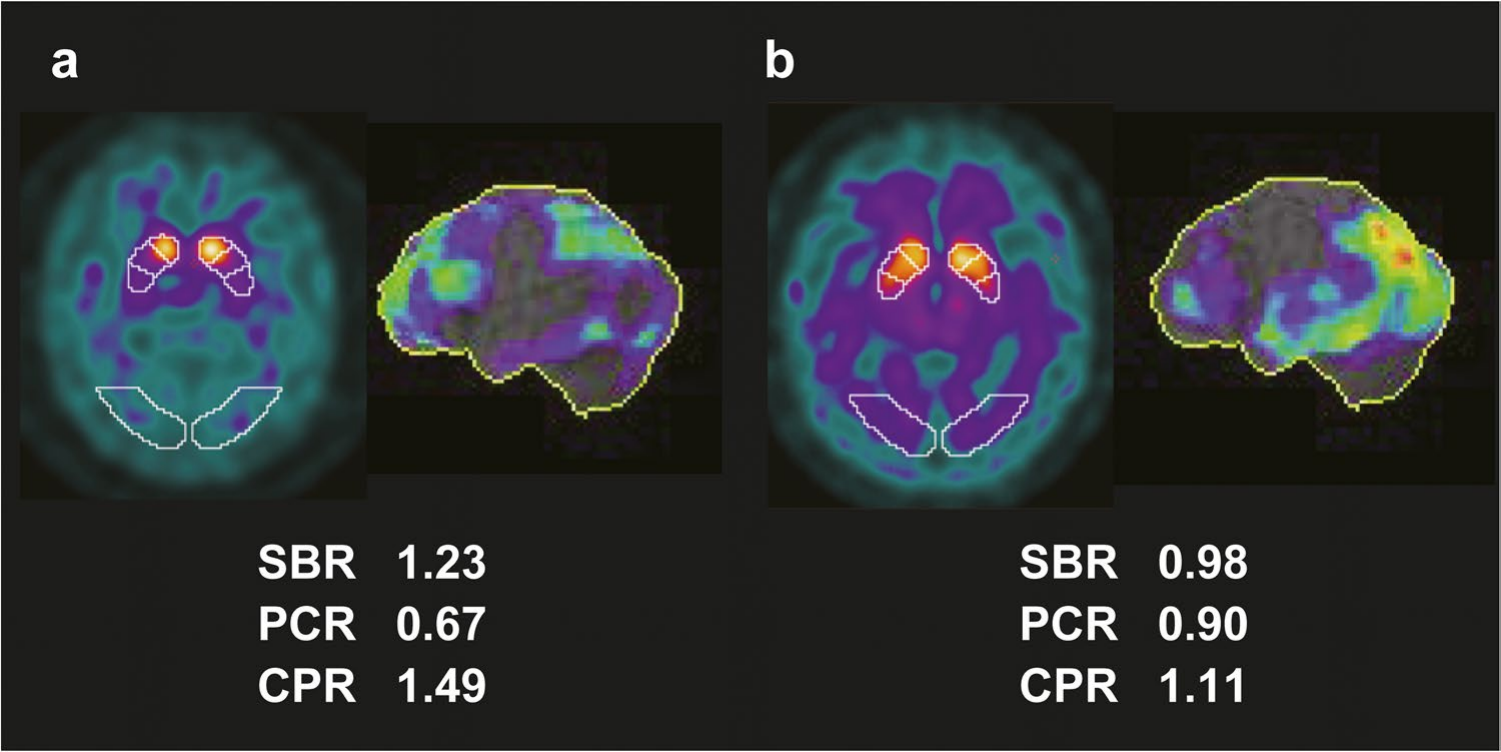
DAT-SPECT and brain perfusion SPECT images of representative cases of PD and DLB. A commercially available software package DaTQUANT was used for VOI-based analysis. The brain perfusion image was visualised with 3D-SSP. a A representative case of PD (aged 66 years, female) shows striatal uptake deterioration, especially in the posterior part. b A representative case of DLB (aged 76 years, male) shows diffuse striatal uptake deterioration and remarkable hypoperfusion in the temporoparietal lobe. DAT, dopamine transporter; DLB, dementia with Lewy body; PD, Parkinson’s disease; SPECT, single-photon emission computed tomography; VOI, volume of interest; 3D-SSP, three-dimensional stereotactic surface projection
